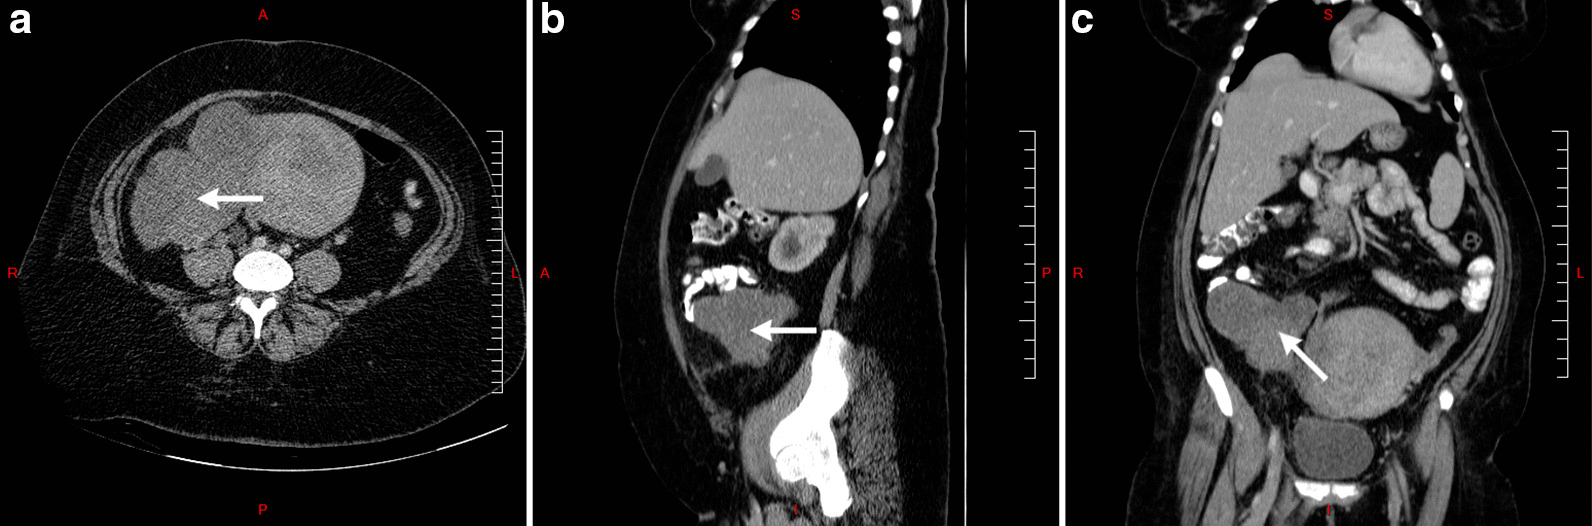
Transverse (a), sagittal (b), and coronal (c) computed tomography scans showing a primary uterine mass as pointed by the white arrow
